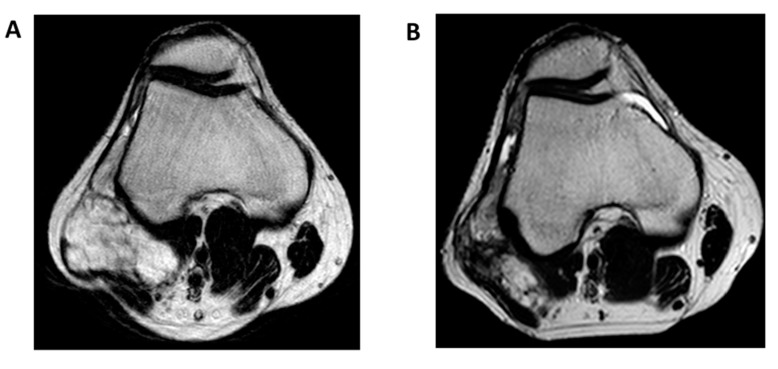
Response to radiation therapy in extraskeletal myxoid chondrosarcoma of the knee. (A) T2-weighted magnetic resonance imaging, axial view, at baseline; (B) T2-weighted magnetic resonance imaging, axial view, after external beam radiotherapy at a total dose of 50 Gy in 25 fractions.
